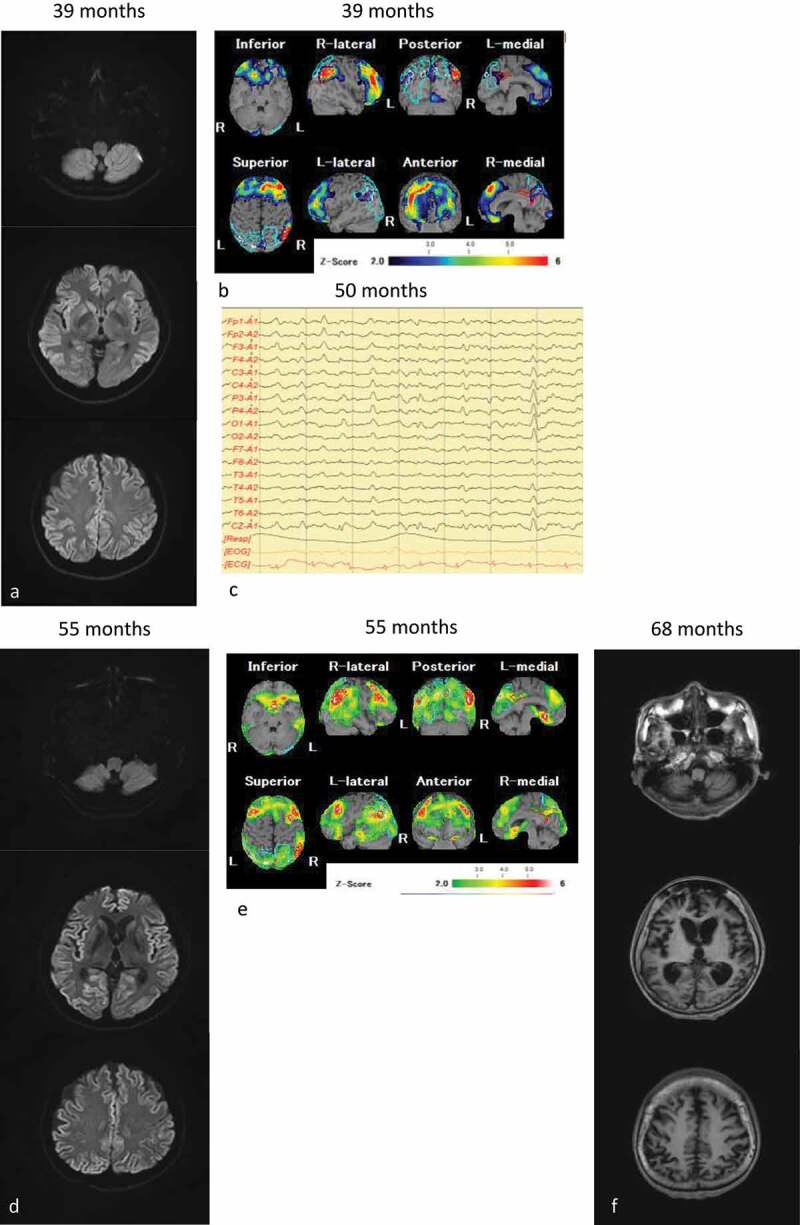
MRI, single photon emission computed tomography (SPECT) and electroencephalography of the patient. (a): Diffusion-weighted MRI (DW-MRI), and (b): SPECT images obtained 55 months after onset; (c): Electroencephalography obtained 50 months after onset; (d): DW-MRI, and E: SPECT images obtained 55 months after onset; (f): T1-weighted MRI obtained 68 months after onset. The eZIS analysis of SPECT images revealed decreased regional cerebral blood flow (rCBF). A higher Z-score indicated a lower rCBF. A Z-score of 2 to 6 is indicated by the green or black-to-red (lower rCBF) colour gradient. Panel A shows the bilateral hyperintense lesions in the frontal, temporal, and parietal cortices, and the basal ganglia. Panel B depicts the decreased rCBF in the frontal and parietal lobes. Panel C depicts the periodic sharp wave complexes. Panel D depicts bilateral hyperintensity in all cortices, and panel E shows preserved rCBF in the brainstem. Panel F depicts progressive cerebral atrophy, but no brainstem atrophy
